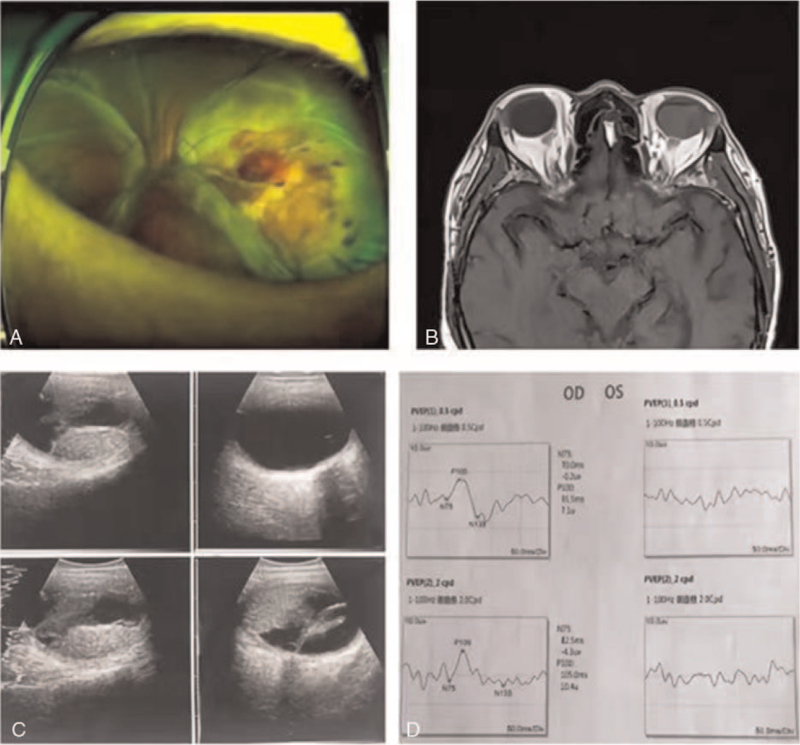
A) Three choroidal masses at the posterior pole of the left ocular fundus, macular involvement with subretinal fluid, without retinal tears, which appeared to represent retinal detachment. B) Solid homogenous mass rich in internal blood flow with exudative retinal detachment. C) The space-occupying lesions in the left eyeball appeared isointense on T1-weighted images and hypointense on T2-weighted images. D) There was no effective waveform at the peak of P100 in the left eye.
